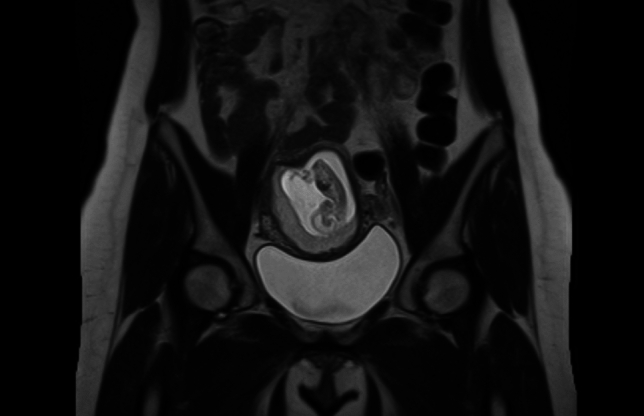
Follow-up MRI image taken while the patient was pregnant
